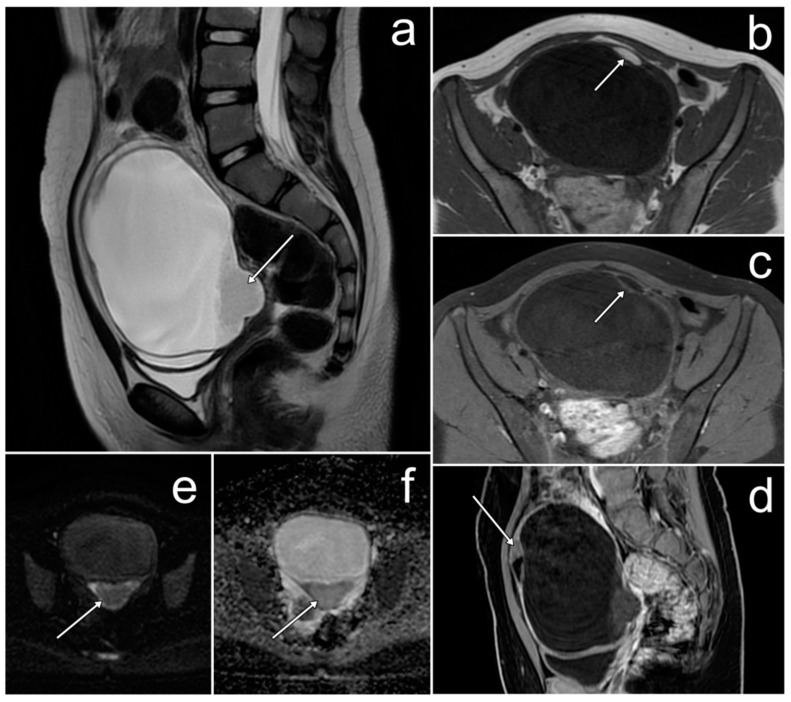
An 11-year-old girl with a giant cystic teratoma of the right ovary (a–f). Typical features of the lesion are present—a gravitationally arranged level of keratinoid material, which shows peripheral diffusion restriction (a,e,f, arrows), a Rokitansky nodule that poorly enhances after the administration of contrast agent (d, arrows), and a fatty element in the wall (b,c, arrows). (a)—FSE/T2; (b)—DIXON/T1 InPhase; (c)—DIXON/T1 WATER; (d)—post-contrast LAVA/T1 WATER; (e)—DWI; (f)—ADC map.
